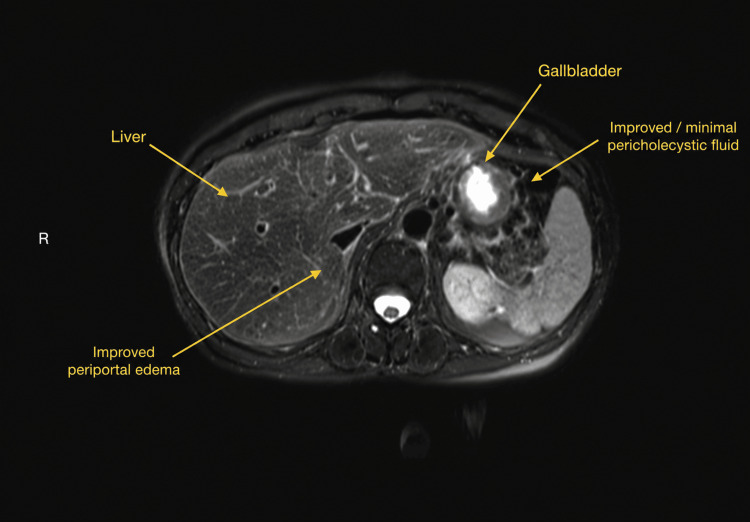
Follow-up axial T2-weighted fat-suppressed MRI demonstrating marked interval resolution of periportal edema and minimal residual pericholecystic fluid following antiviral therapy for disseminated HSV-2 infection. *Abbreviations:* MRI, magnetic resonance imaging; HSV-2, herpes simplex virus type 2.
